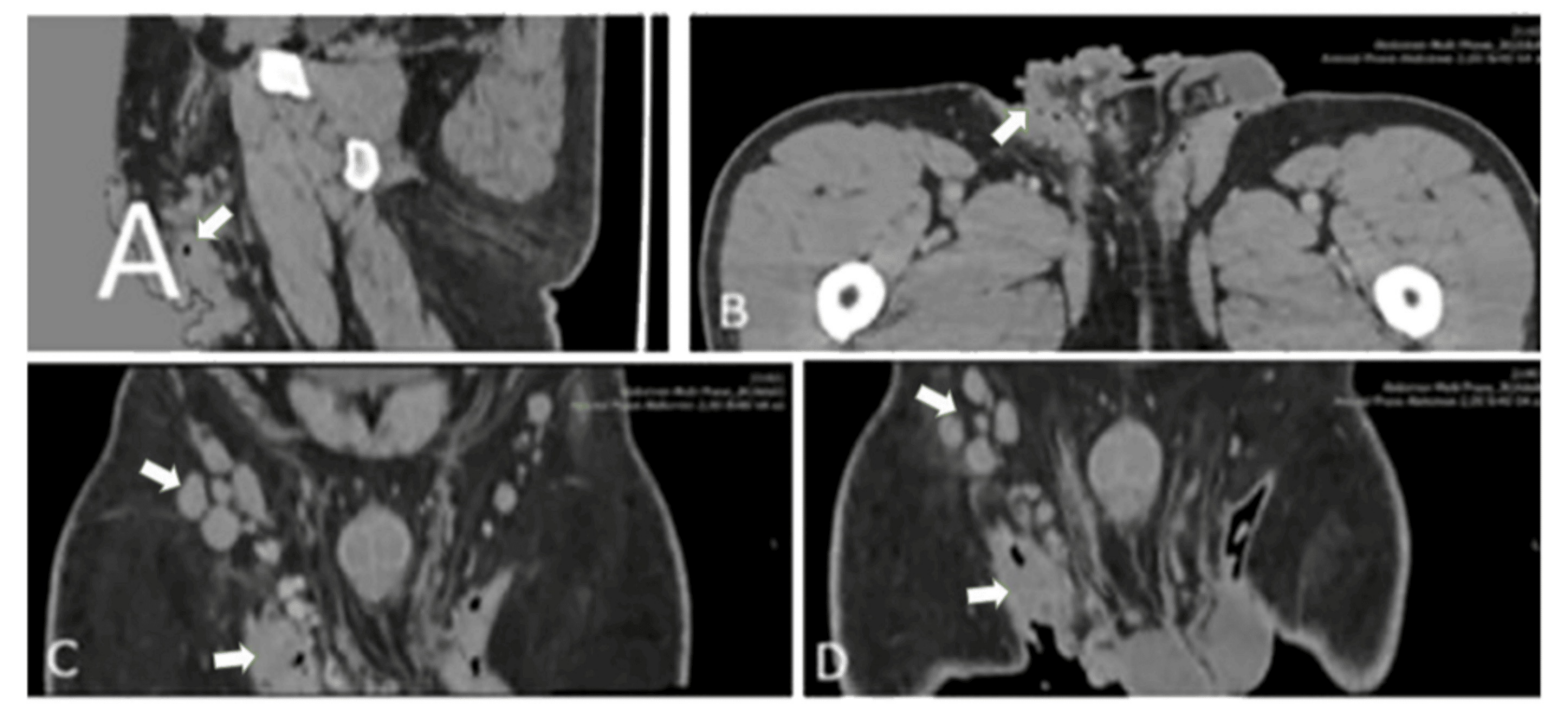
CT images. (A) Sagittal image. (B) Traverse image. (C) Coronal image. (D) Coronal image. Significant irregular thickening with lobulated margins in the perineum, as well as in the scrotum, more prominently on the right, with multiple enlarged lymph nodes.
